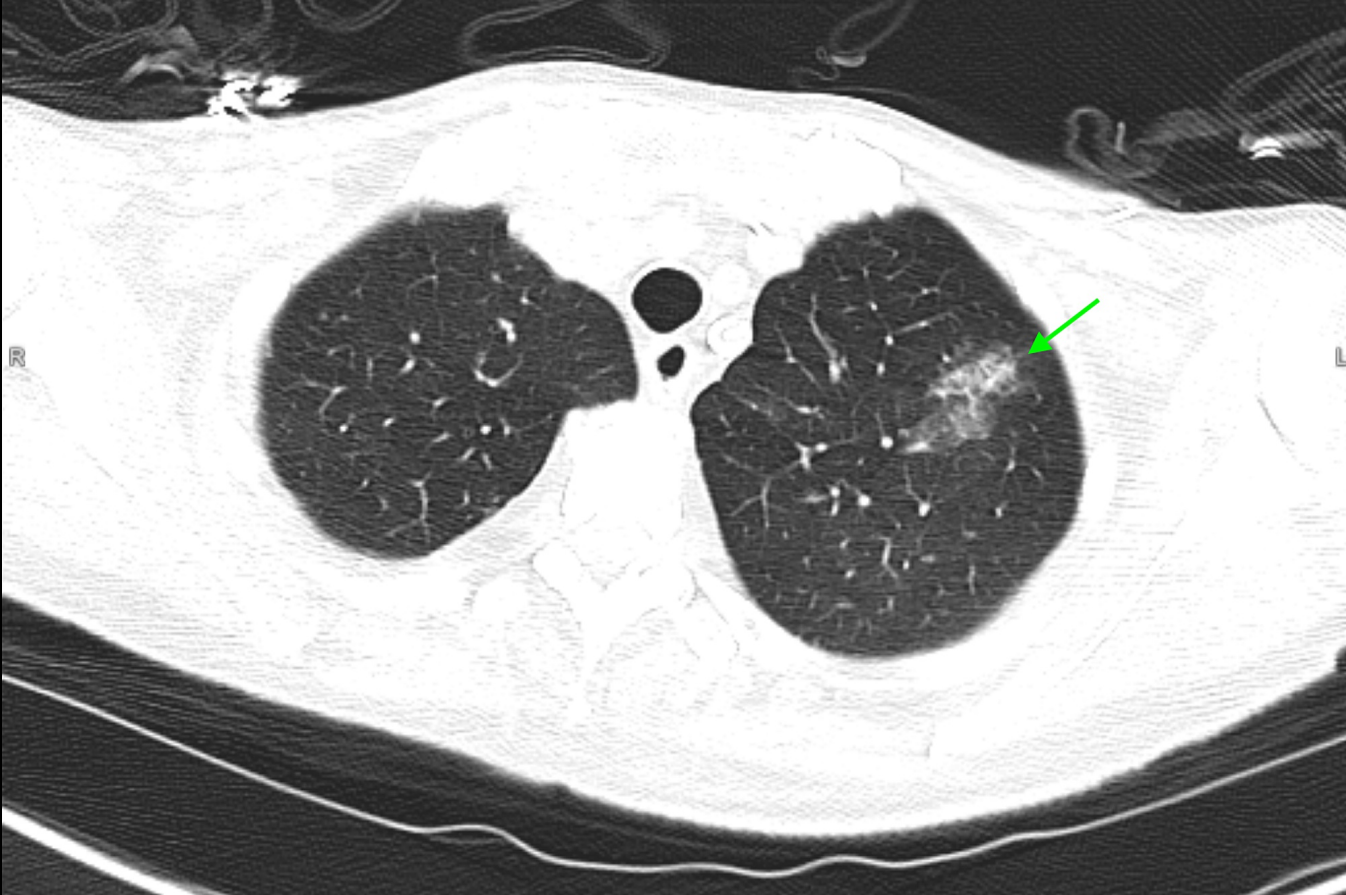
CT chest. CT chest showing a focal ground-glass attenuation in the left upper lobe of lung.
